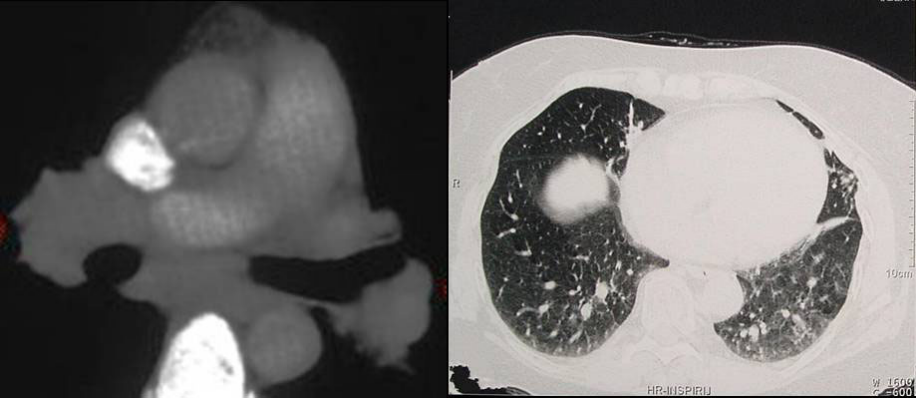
Thorax CT with hilar lymph nodes enlargement (a) and reticular interstitial pattern with granulomas of both lower lobus (b).
